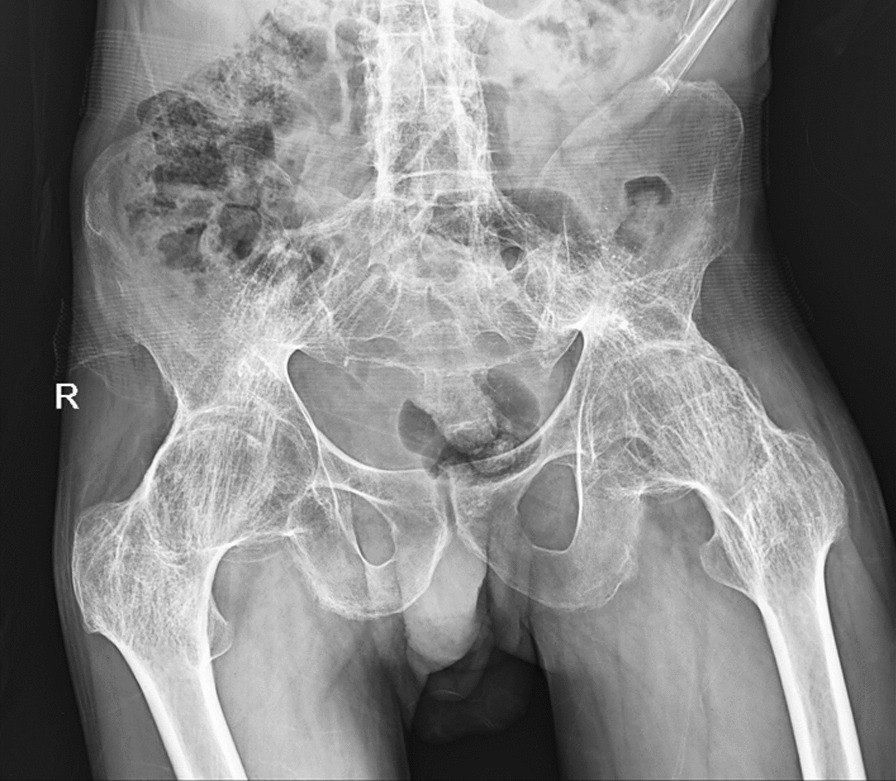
Initial pelvic plain radiograph revealed bilateral fused hips and bamboo spine
